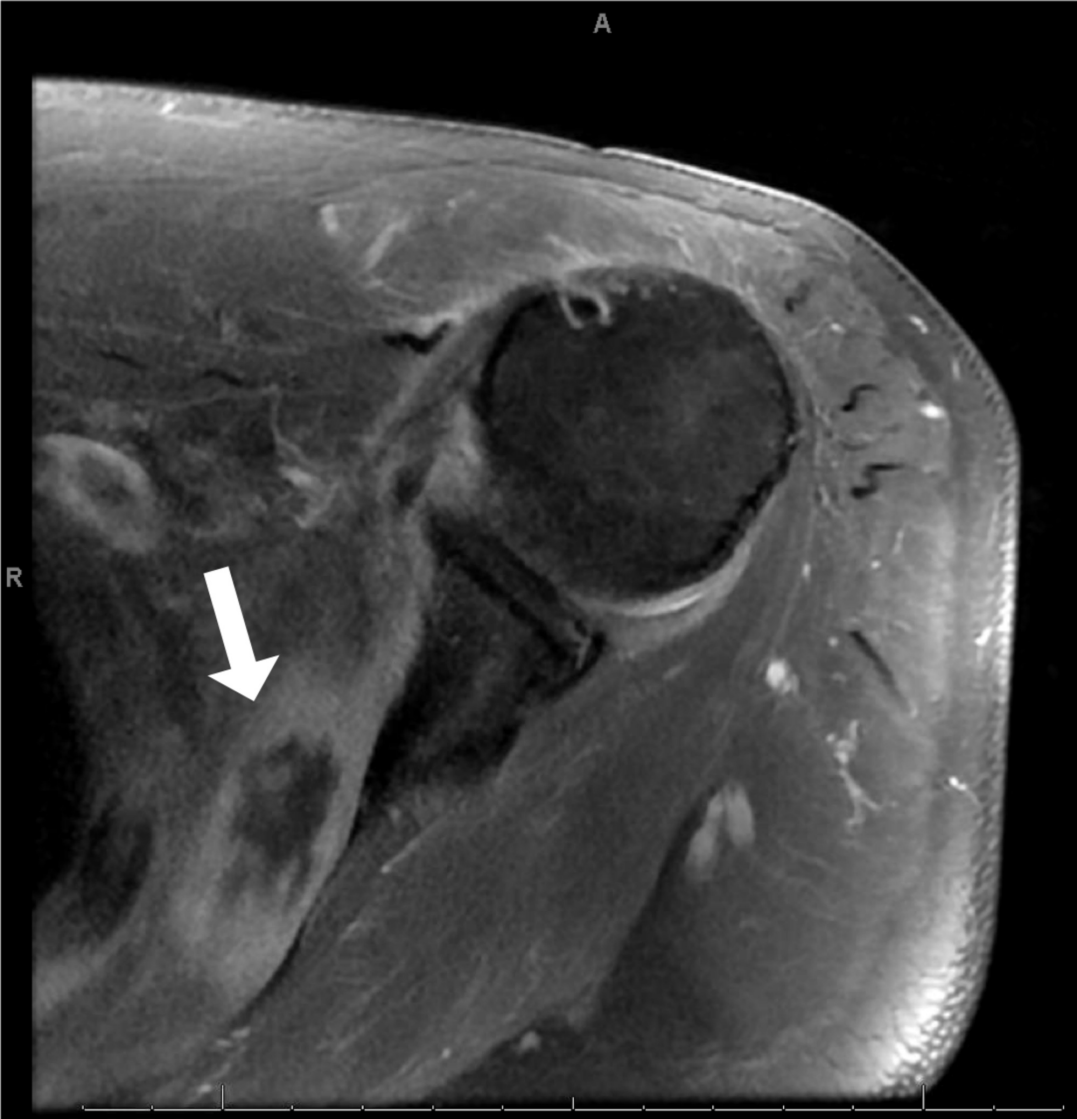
Left axial T1-weighted MRI showing subscapularis lesion with irregular peripheral edema and non-enhancing central core suggestive of myonecrosis. MRI, magnetic resonance imaging
